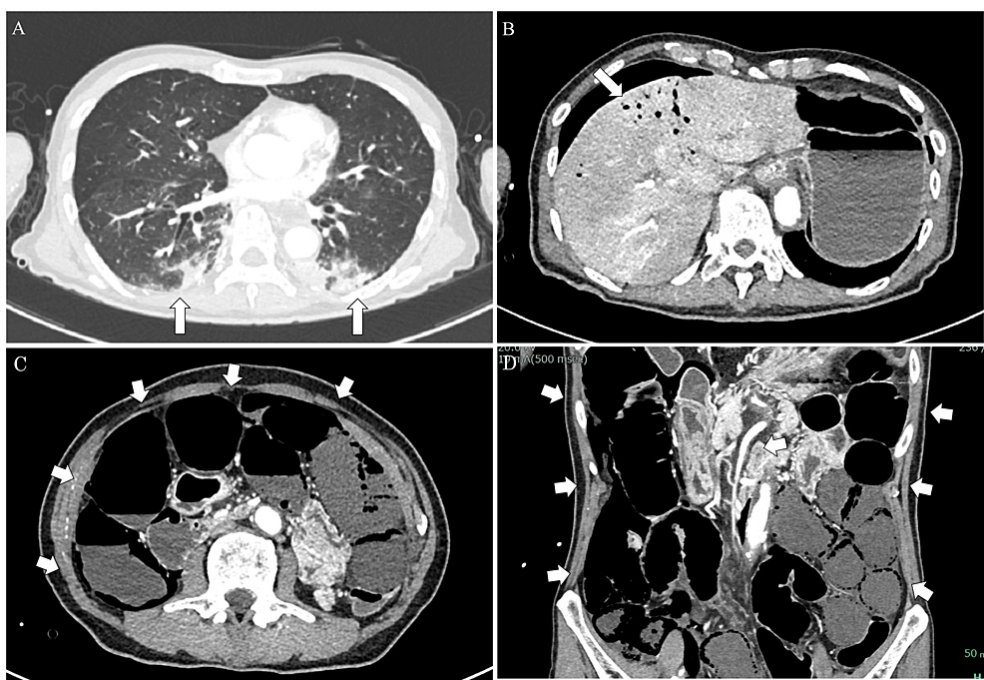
Contrast-enhanced CT image at the time of NOMI onset. (A) Patchy infiltrates are observed in the bilateral lungs. (B) Intrahepatic portal vein gas is found in the liver. (C) Extensive intestinal emphysema associated with intestinal necrosis is observed. (D) There is no occlusion of the superior mesenteric artery due to a thrombus or other reasons. CT: computed tomography, NOMI: nonocclusive mesenteric ischemia
